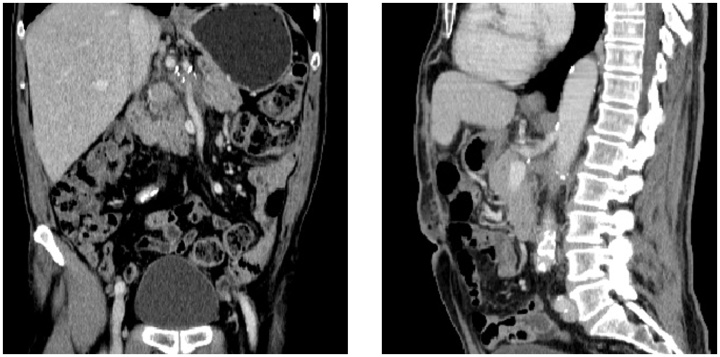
Abdominal CT at three months showed permeability of the celiac trunk and the superior mesenteric artery.
